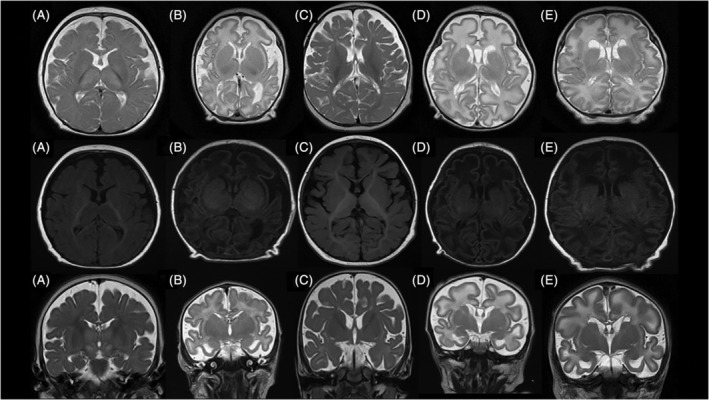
Brain MRI features. First row: axial T2 sequence; second row: axial T1 sequence; third‐row: coronal T2 sequence. A, Patient A at 2 months almost normal with mild delayed myelination. Increased subarachnoid spaces. B, Patient B at day 3 delayed myelination with temporal cysts and broad gyration with increased subarachnoid spaces and cortico‐subcortical atrophy. C, Patient C at 10 months mild delayed myelination, increased subarachnoid spaces, and cortico/subcortical atrophy. D, patient D at 1‐month broad gyration, delayed myelination, temporal cysts increased subarachnoid spaces, and cerebral atrophy. E: patient E at day 3 broad gyration, delayed myelination, temporal cysts, increased subarachnoid spaces, and cortico‐subcortical atrophy
